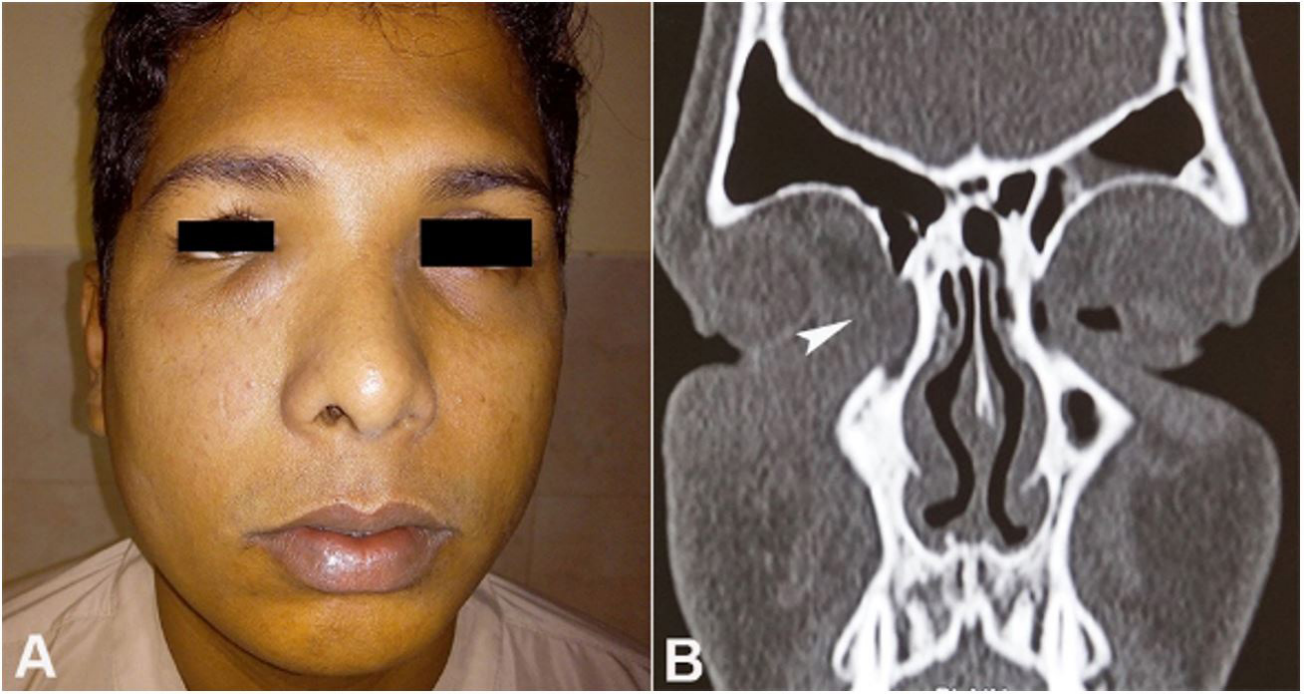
A – External view of the patient’s face showing the swelling below the medial canthus of the right eye; B – Non-contrast CT scan (Coronal plane) revealed a soft tissue density in the lacrimal sac without bony erosion (arrowhead).
